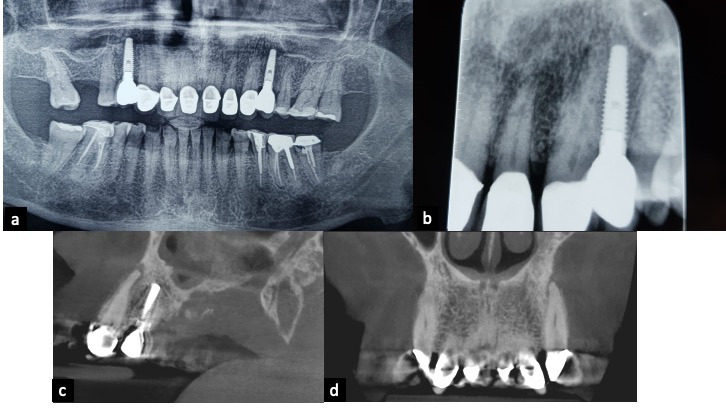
a) Radiografía panorámica; b) Radiografía periapical, diente 23; c) Imagen de CT, corte sagital, diente 23; d) Imagen de CT, corte coronal, diente 23. Imágenes diagnósticas influyen en la decisión de tratamiento.
